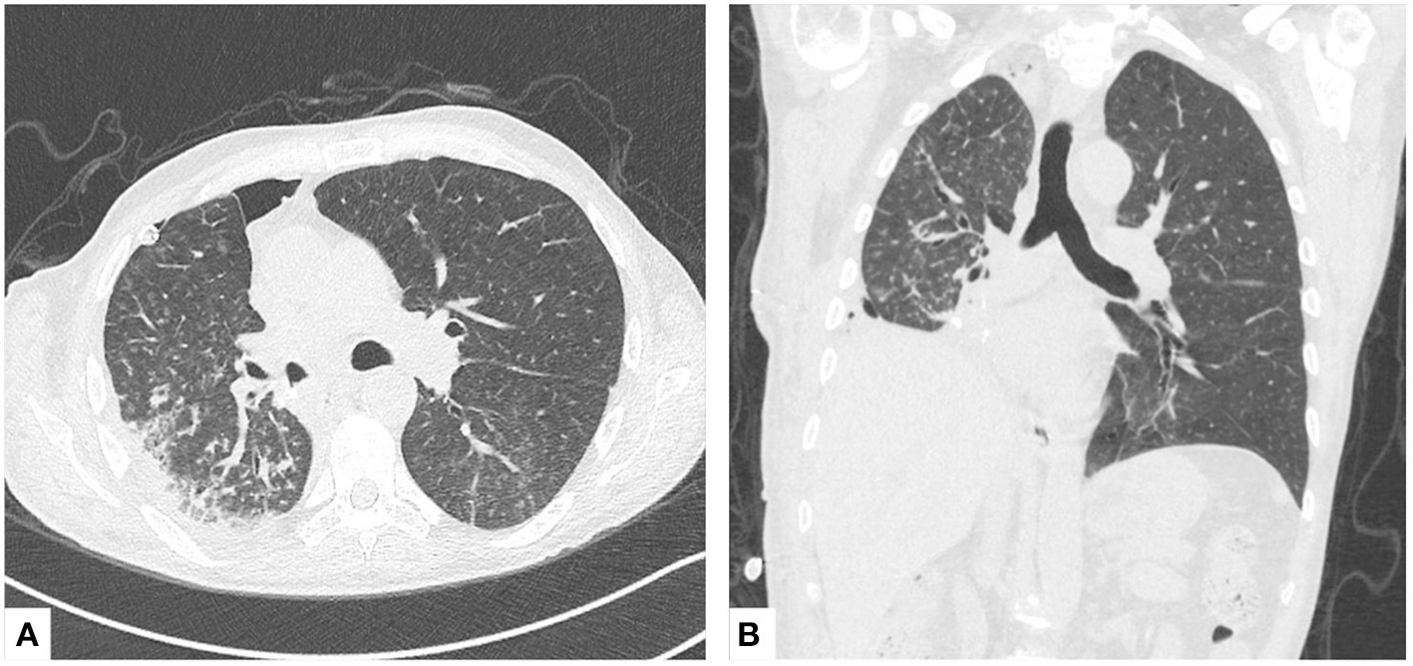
Postoperative CT scan. (A) Axial and (B) coronal views of chest CT scans 1 month after the inferior bilobectomy.
